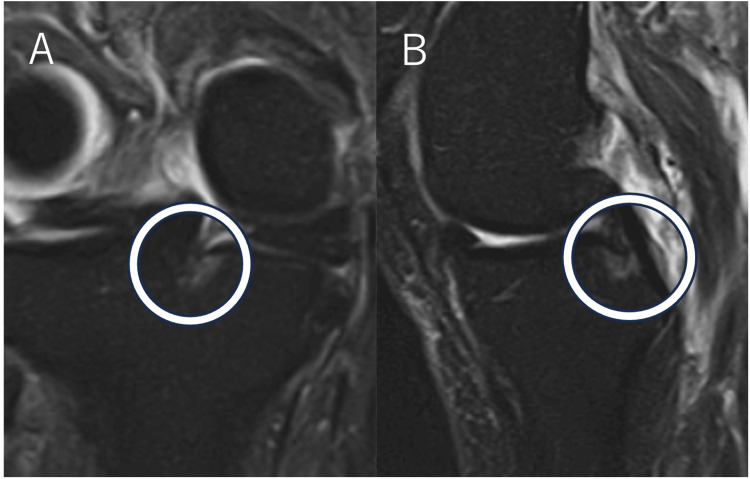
MRI findings of PSCL The STIR (TR 3,300 ms/TE 30 ms, 1.5 Tesla) images are positive findings for PSCL on coronal (A) and sagittal (B) images. MRI: magnetic resonance imaging; STIR: short T1 inversion recovery; PSCL: posterior shiny corner lesion
